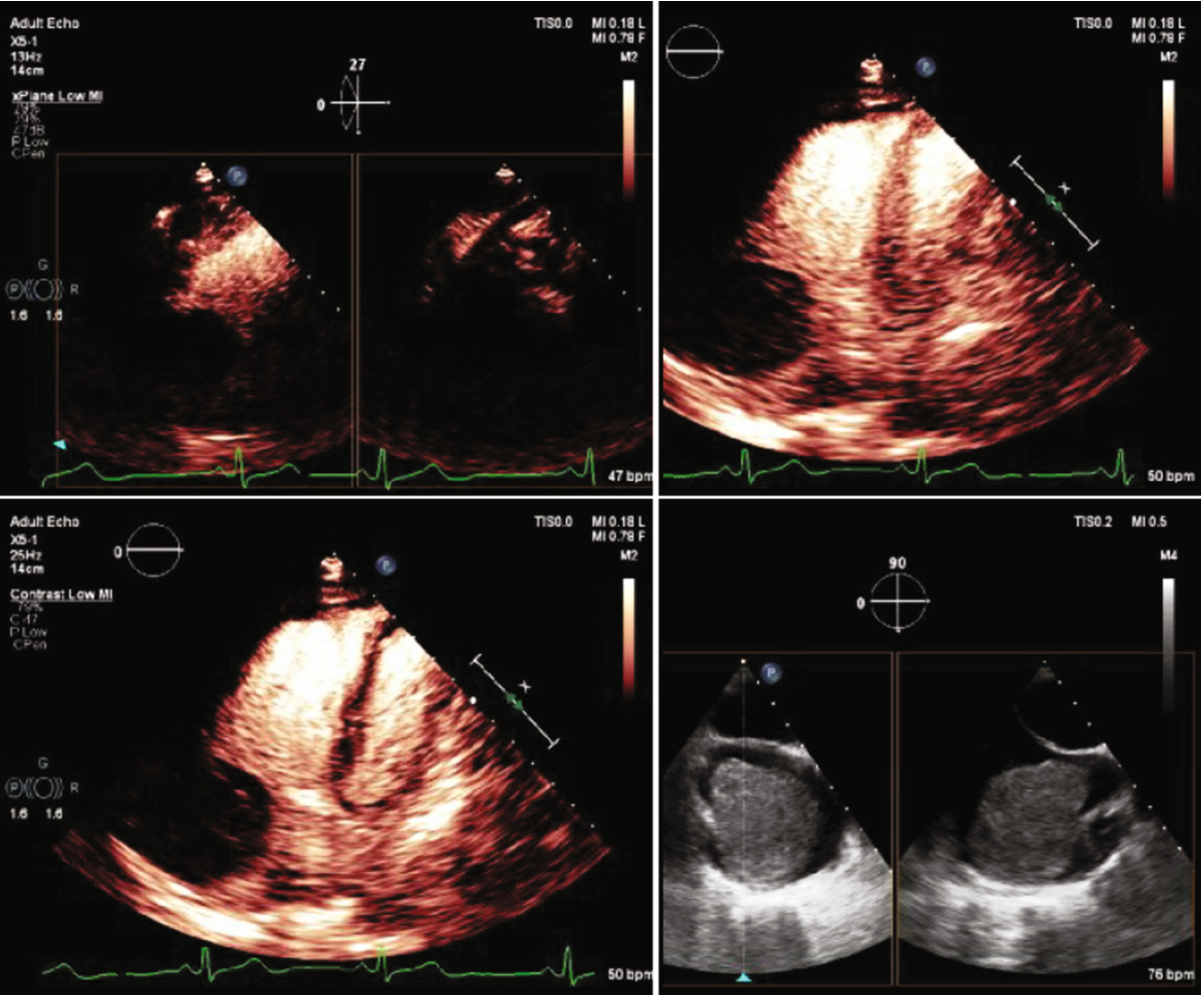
Transesophageal echocardiography showing echogenic right atrial mass with a possible noted stalk.
